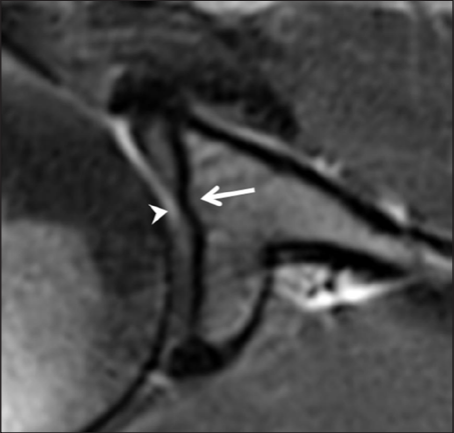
Tubercle of Assaki. Axial fat-saturated PD-weighted MR image shows focal elevation of the subchondral bone (arrow) in the mid third of the glenoid with focal thinning of overlying cartilage (arrowhead).
